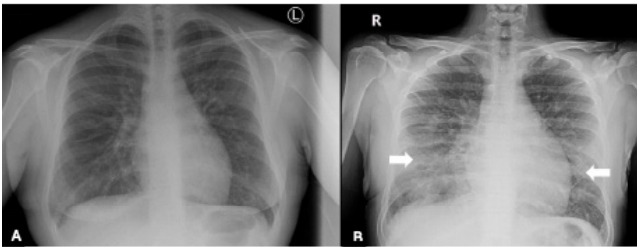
Chest radiograph on (A) initial presentation and (B) following the development of respiratory distress after intravenous hydration, demonstrating bilateral interstitial infiltrates (arrows).
